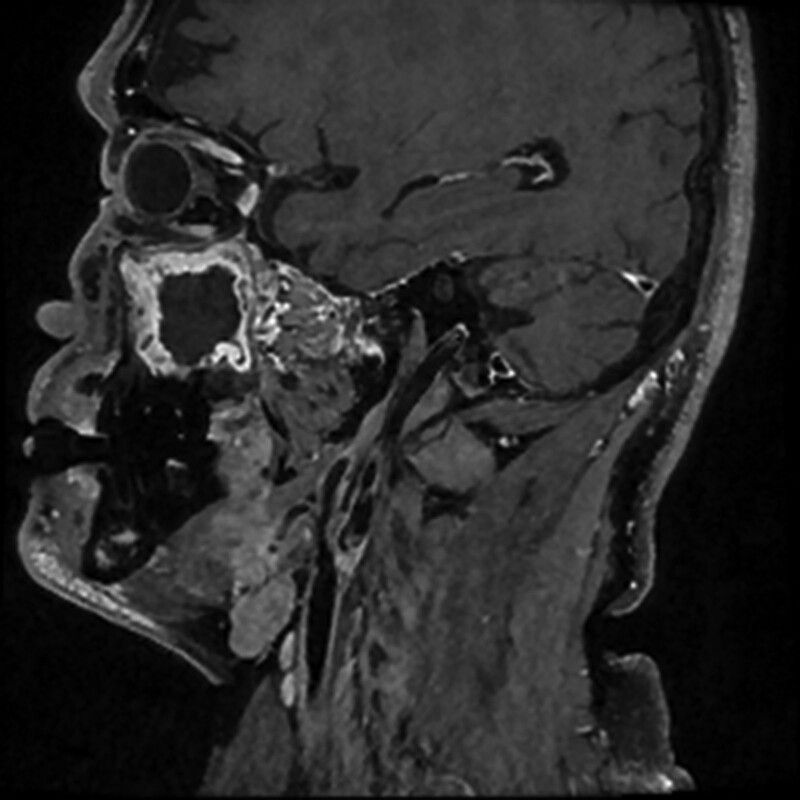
Magnetic resonance imaging showing right proximal internal carotid artery stenosis and the high position of the carotid artery bifurcation.
